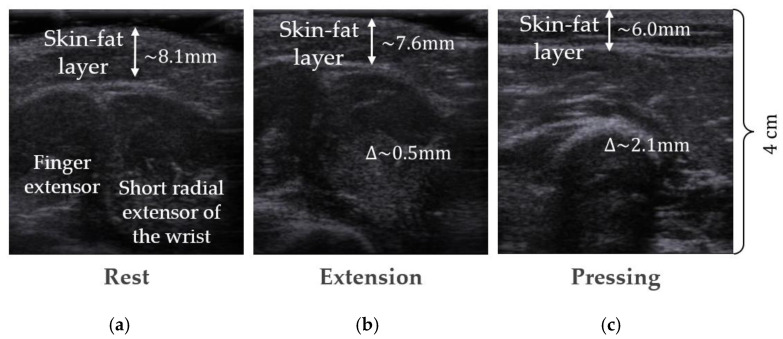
Example of transverse ultrasound images of internal structures of the forearm: (a) at rest; (b) when performing a wrist extension; (c) with an increase in the pressing force of the ultrasonic sensor to the skin surface.
